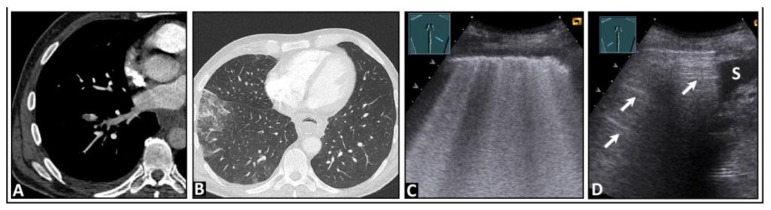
A patient experiencing right-sided, respiration-dependent thoracic pain due to a right-sided central pulmonary embolism. (A,B) The chest computed tomography scan shows bilateral pulmonary embolism, more pronounced on the right, especially affecting the lower lobe, with the onset of infarction pneumonia in the right lower lobe. (C) On lung ultrasound, there are multiple confluent B-lines on the right side, indicating a “white lung” pattern with a rough lung surface. (D) The left side displays a normal lung surface with A-lines (arrows); S = spleen.
